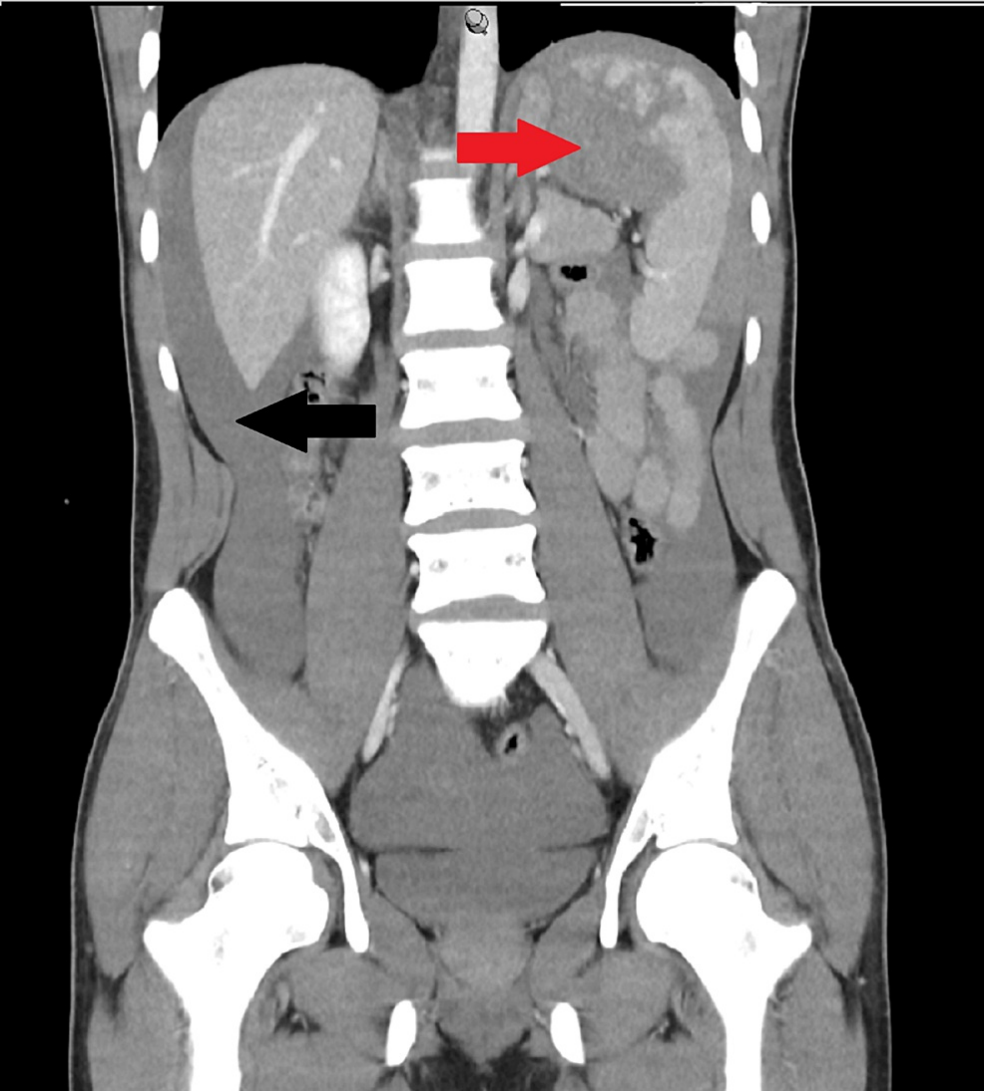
Abdominal CT showing significant haemoperitoneum and Grade IV splenic injury involving the upper pole Red arrow: Grade IV splenic injury producing >25% devascularisation of the spleen Black arrow: Significant haemoperitoneum
